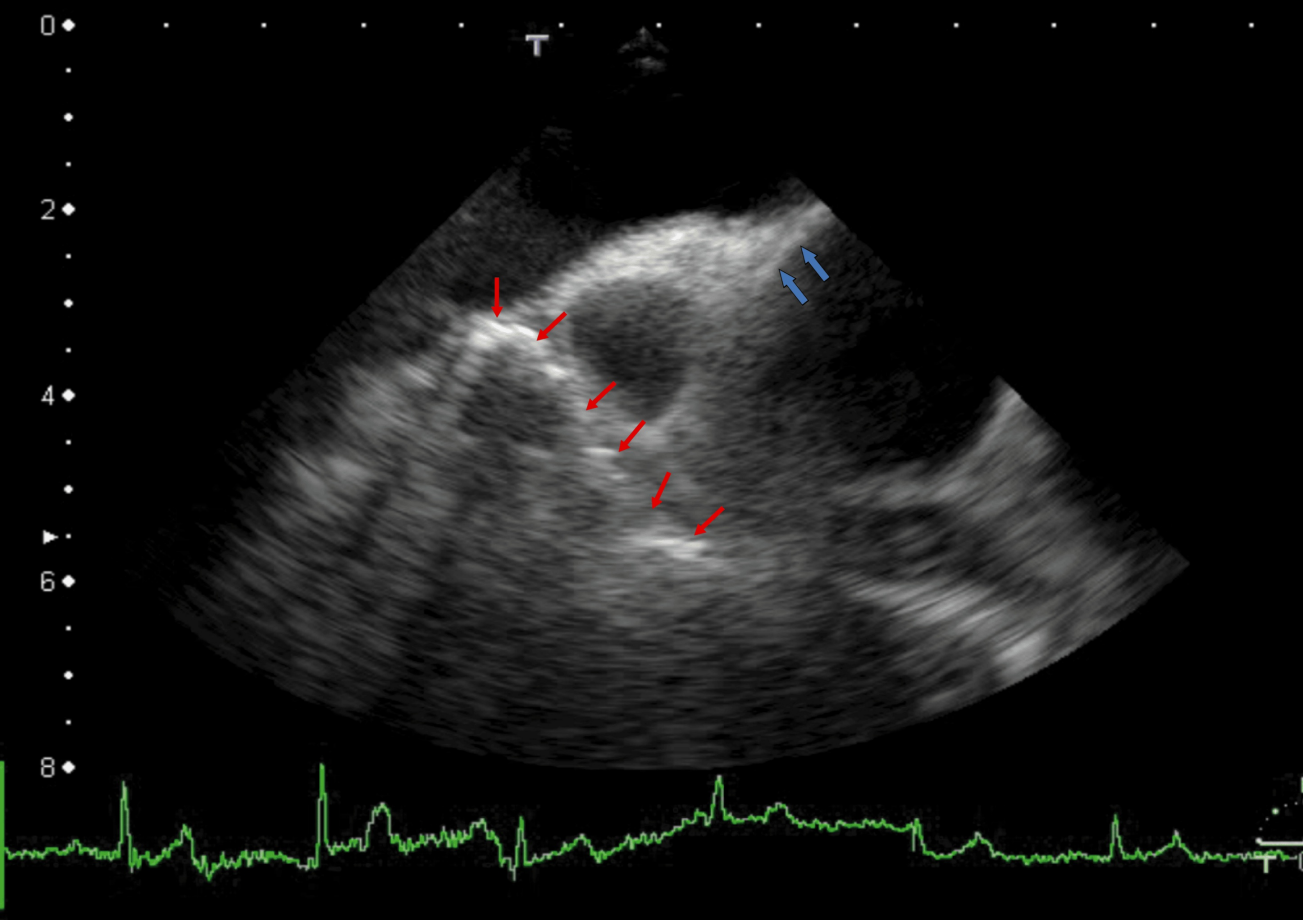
TEE image showing resolution of some linear vessel thrombi after six months of standard-dose apixaban treatment. After six months of standard-dose apixaban treatment, TEE revealed that some linear vessel thrombi had resolved (red arrows). Only right-sided thrombi exhibited high-echo-intensity shadows. Some areas of the AAo wall showed a normal structure (blue arrows). The cleft in the low-echo-intensity areas had disappeared. TEE: transesophageal echocardiography; AAo: ascending aorta
